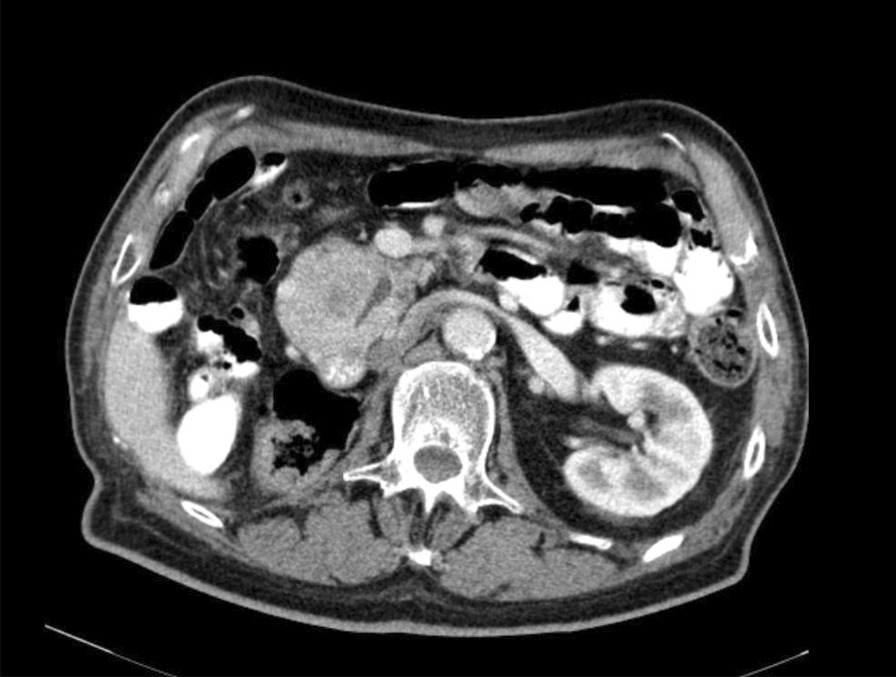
Axial contrast-enhanced computed tomography demonstrating tumor of the pancreatic head with stenosis of the main pancreatic duct
